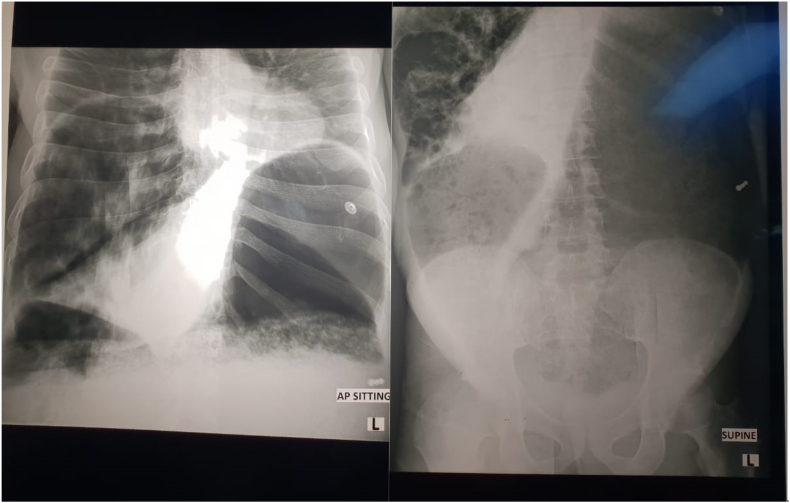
Abdominal X-Ray - Distended pelvic stomach and right upper quadrant cystic appearance.
